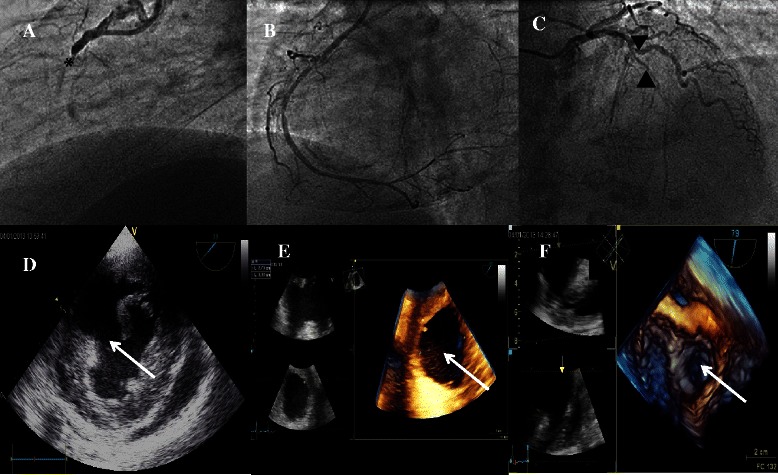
Preoperative imaging. (A) Coronary angiography revealed an acute occlusion (*) of the right coronary artery; (B) revascularization with percutaneous coronary intervention; (C) chronic sub-occlusive lesion of the left anterior descending artery (▲) and a 90% stenosis in the ostium of the first diagonal artery (▼) were also revealed; (D) transesophageal echocardiography showed a muscular ventricular septal defect (VSD); (E) real-time 3-D transesophageal echocardiography depicted a large septal defect measured 38×27mm; (F) the defect was further located in the anterior middle portion of the muscular ventricular septum in the reconstructed image with a view from the left ventricle. The white arrows indicate the location of the VSD.
